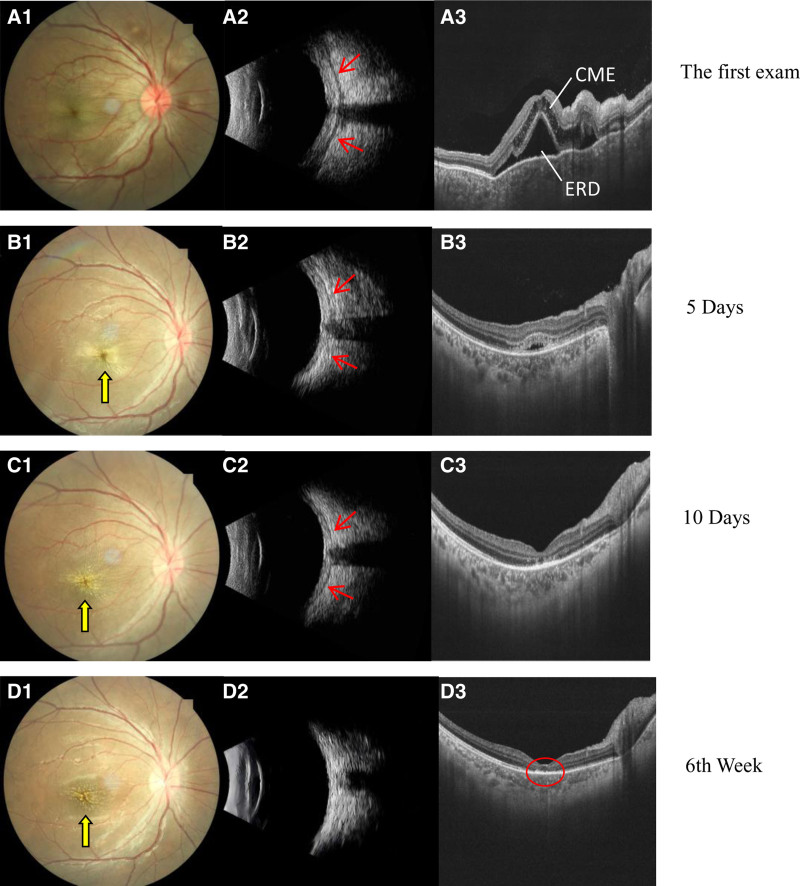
Fundus, B-scan ultrasound, and optical coherence tomography on admission, and after 5-day, 10-day, and 6-week therapy in the right eye. (A1) Right fundus showing disc edema and tortuous vessels. (B1, C1) Subretinal exudates at the macula occurring during treatment. (D1) Fundus exudates remaining at the macula after 6-week therapy. (A2) B-scan ultrasound of the right eye showing a prominent T-sign. (B2, C2) The eye wall gradually thinning after therapy. (D2) Resolution of the T-sign. (A3) Optical coherence tomography showing cystoid macular edema (CME) and exudative retinal detachment (ERD) in the right eye. (B3, C3) The macular edema is reducd, and the subretinal fluid is absorbed after therapy. (D3) Retinal structure disordering, ellipsoid zone and the interdigitation zone continuity interruption.
